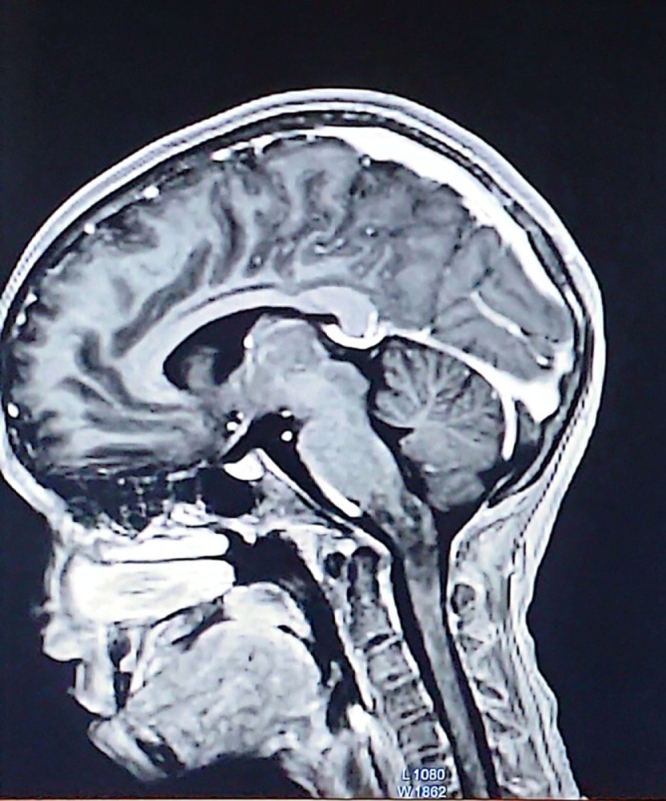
Non-enhancing hyperintensity in medulla and proximal aspect of cervical spine (T1-weighted image).
